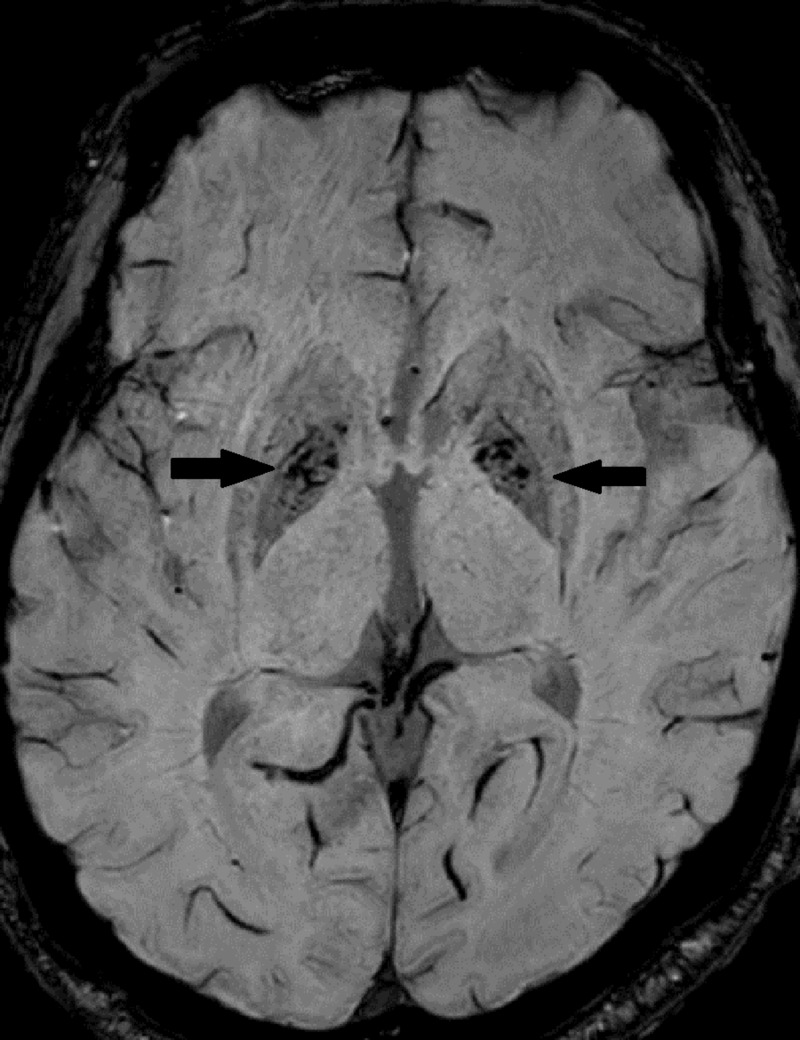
GRE: Transverse Gradient Echo / SWI Susceptibility Weighted Imaging Transverse MRI GRE sequence shows symmetric decreased intensity (dark signal) in the basal ganglia (see arrows). This represents iron accumulation in the basal ganglia. GRE: gradient echo; SWI: susceptibility weighted imaging.
